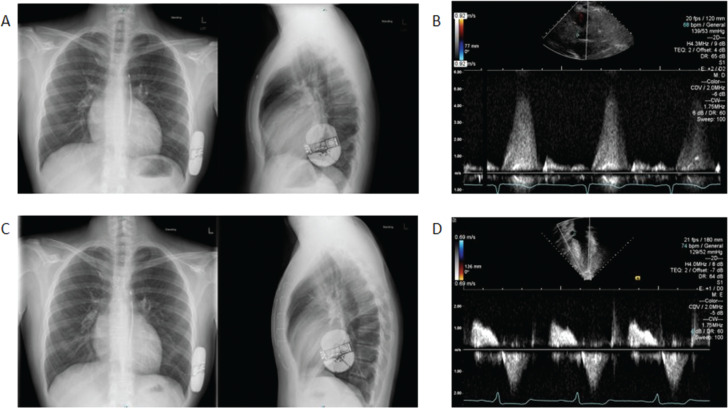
A: Anteroposterior and lateral chest X-rays just prior to sternotomy showing device placement with a PRAETORIAN score of 60 points, suggesting a low risk for conversion failure.^13^
